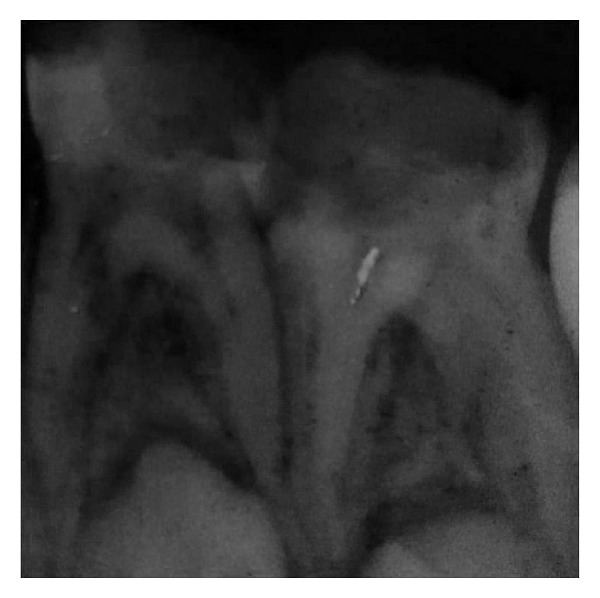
Preoperative radiograph showing advanced dental caries in left mandibular first and second molars.
